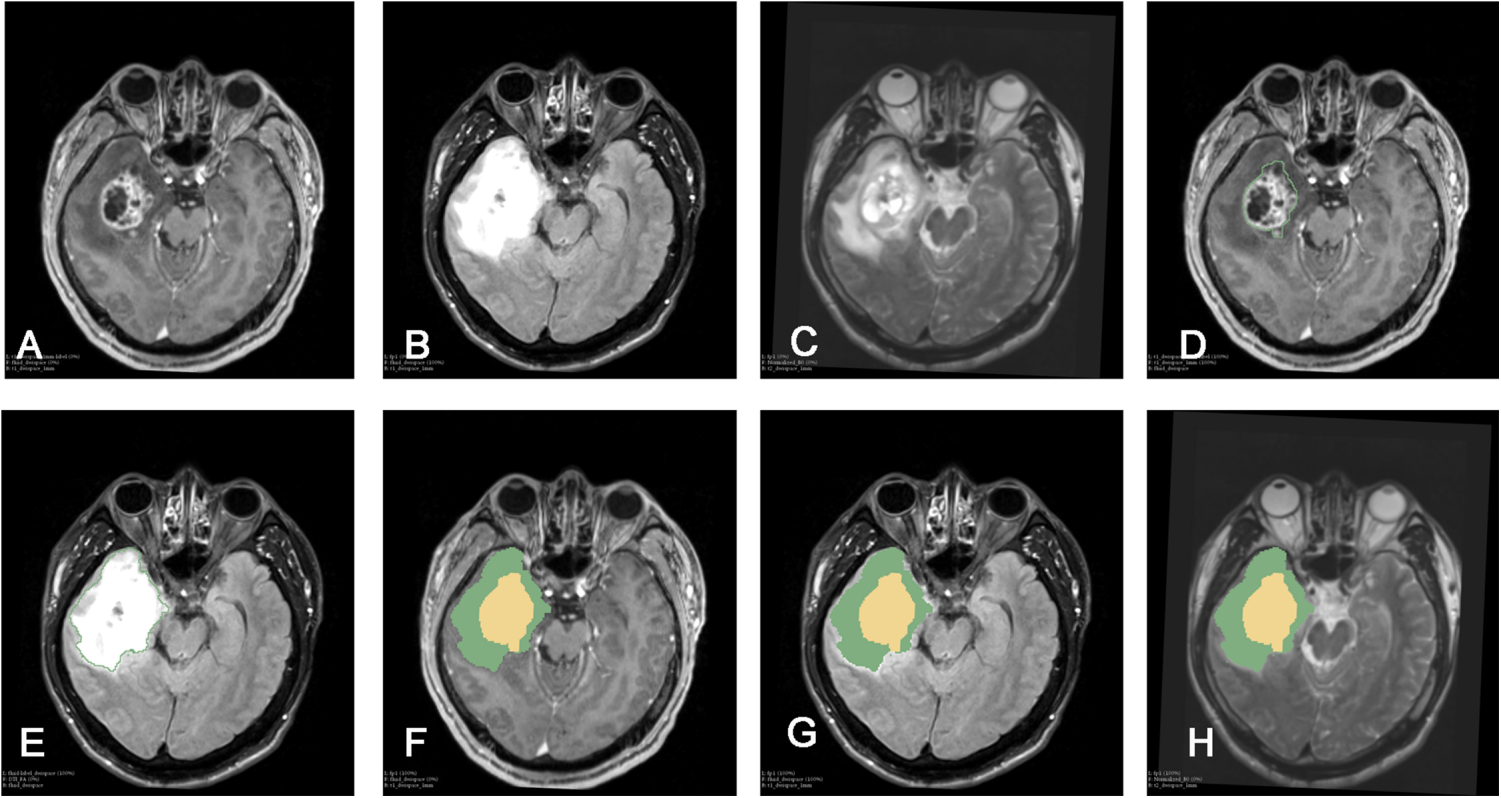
Procedure of tumor segmentation of Case. Case: 43 years old, male, headache for more than half a month, MRI showed right temporal lobe lesions, after surgical resection, pathological examination reported for WHO IV glioblastoma, IDH wild type. As shown in the figure, patient MRI T1enhancement, T2 and T2-FLAIR were shown, after importing into 3D Slicer software, Editor module was used to segment the tumor: (A) T1+C shows tumor enhancement; (B) T2-FLAIR sequence; (C) T2 shows the tumor area; (D) Segmentation of the tumor-enhanced part according to the T1 enhancement sequence in the Slicer software is shown as Label-1; (E) Segmentation of the whole tumor according to the T2-FLAIR is shown as Label-2; (F) T1+C shows Label-3. In the Add Scalar Volume module of Slicer, Label-1 (enhanced part) and Label-2 (tumor whole) are superimposed to obtain the enhanced part (yellow) and the tumor edema part, respectively. (Green) Label-3; (G) T2-FLAIR shows the enhanced part (yellow) and tumor edema (green) after segmentation, respectively; (H) T2 image shows the enhanced part (yellow) and tumor edema, respectively Part (green).
